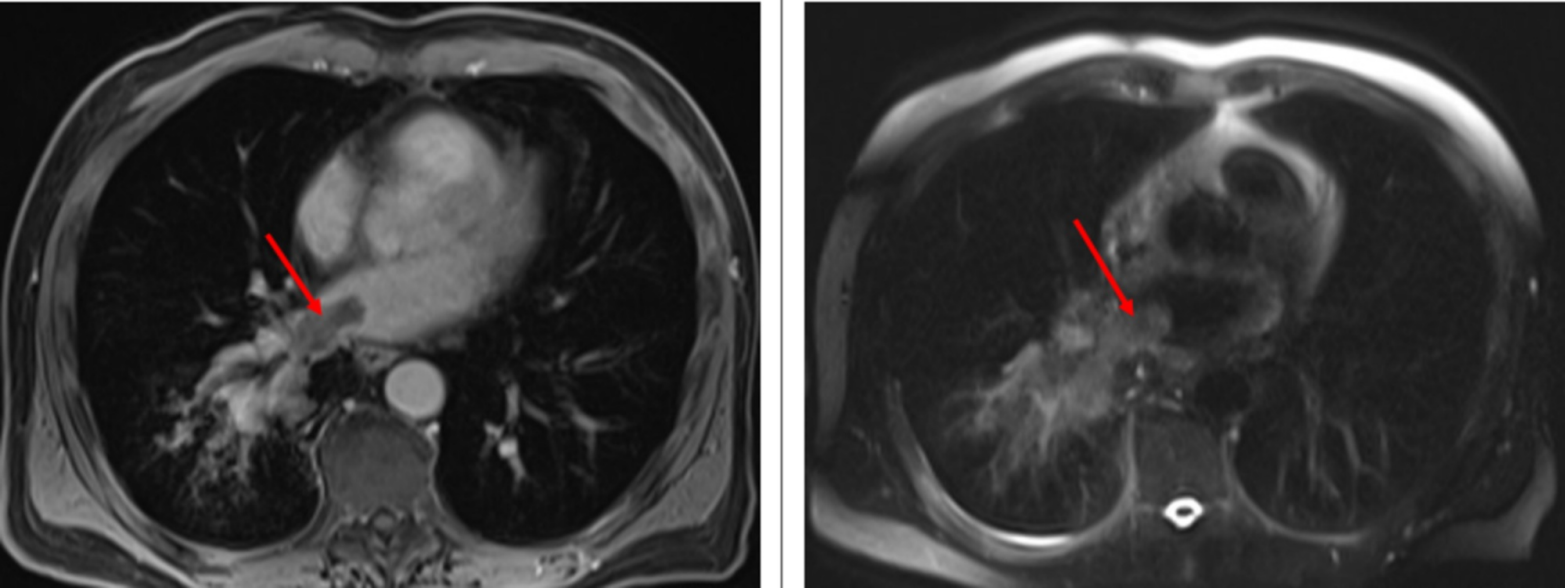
Cardiac MRI, with and without intravenous gadolinium demonstrates a tumor thrombus involving the right inferior pulmonary vein and extending into the left atrium (red arrow).
